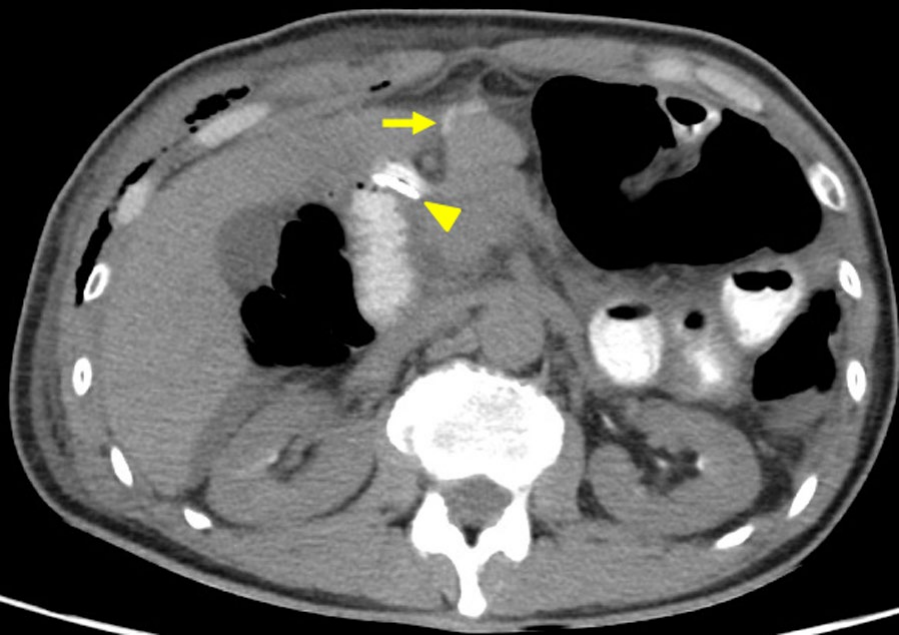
An axial computed tomographic slice on the third postoperative day. Ascites and leakage of oral contrast media are observed in the intraperitoneal cavity. Arrow: the leakage of oral contrast media, Arrowhead: The Duodenal stump
